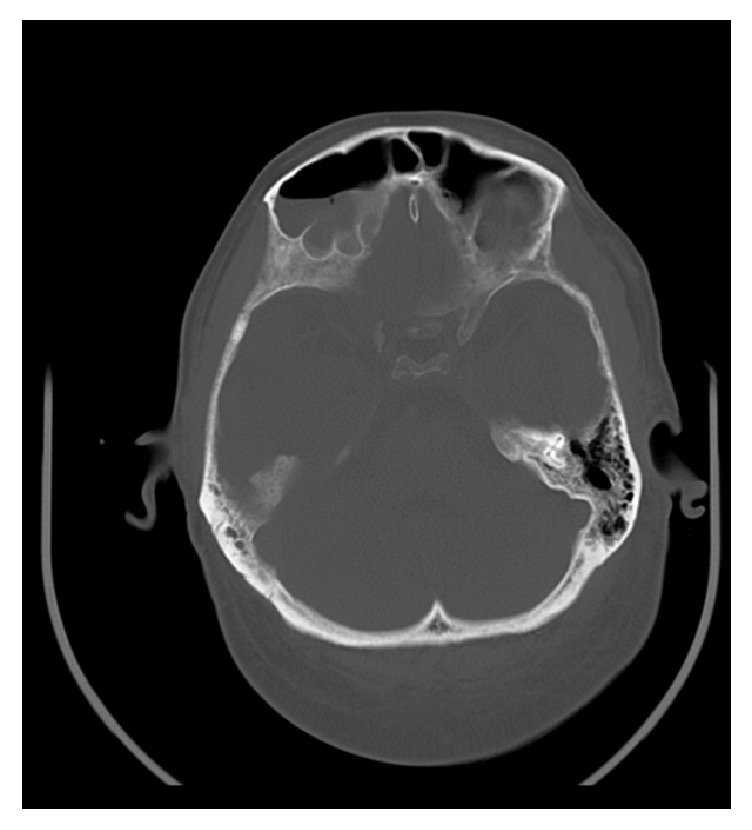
MR imaging of the cranium with air-fluid level in both frontal sinuses.
